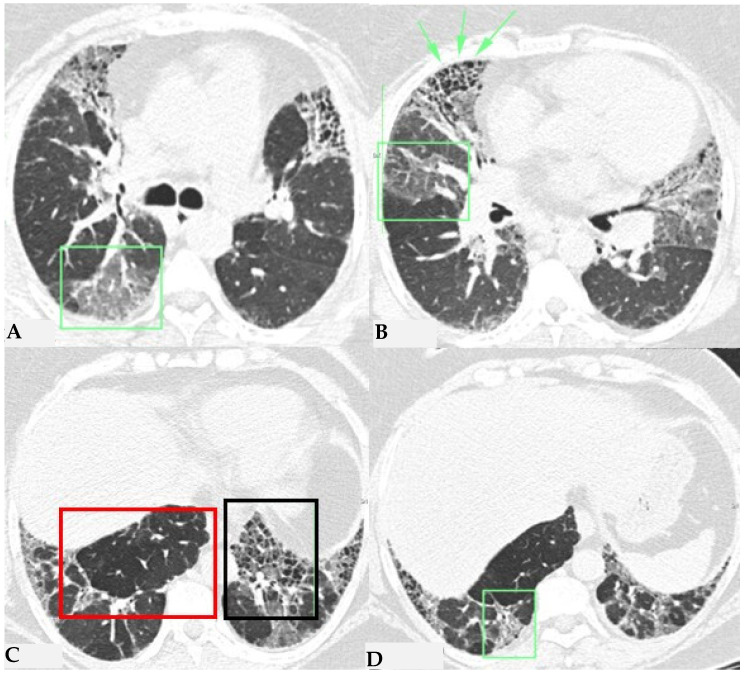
FHP: chest HRCT (A–D). Upper lobes and right lower lobe are characterized by areas of increased GGO density (green box: (A,D)) within which well-defined bronchiectasis and traction bronchiolectasis, indicative of established fibrotic damage, are observed (green arrows: (B)). Lower lobes extensively affected by mixed alterations, expressing damage to small airways with areas of reduced attenuation suggestive of air trapping (red box: (C)), and confirmed fibrotic damage with honeycombing and traction bronchiectasis (black box; (C)). Pattern of FHP.
